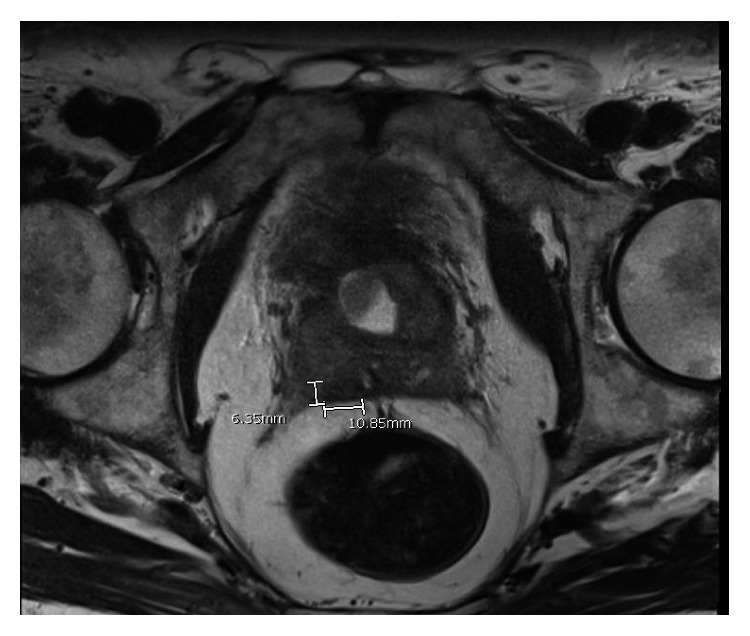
Pelvic MRI after treatment (axial view).
